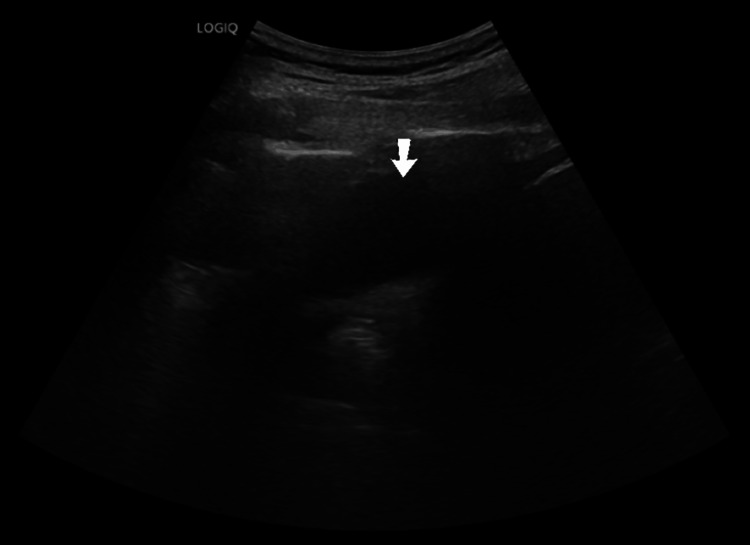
Thoracic ultrasound of right pleural effusion. Thoracic ultrasound demonstrating a large anechoic fluid collection in the right pleural space (arrow), without internal septations, corresponding to the right pleural effusion before aspiration.
